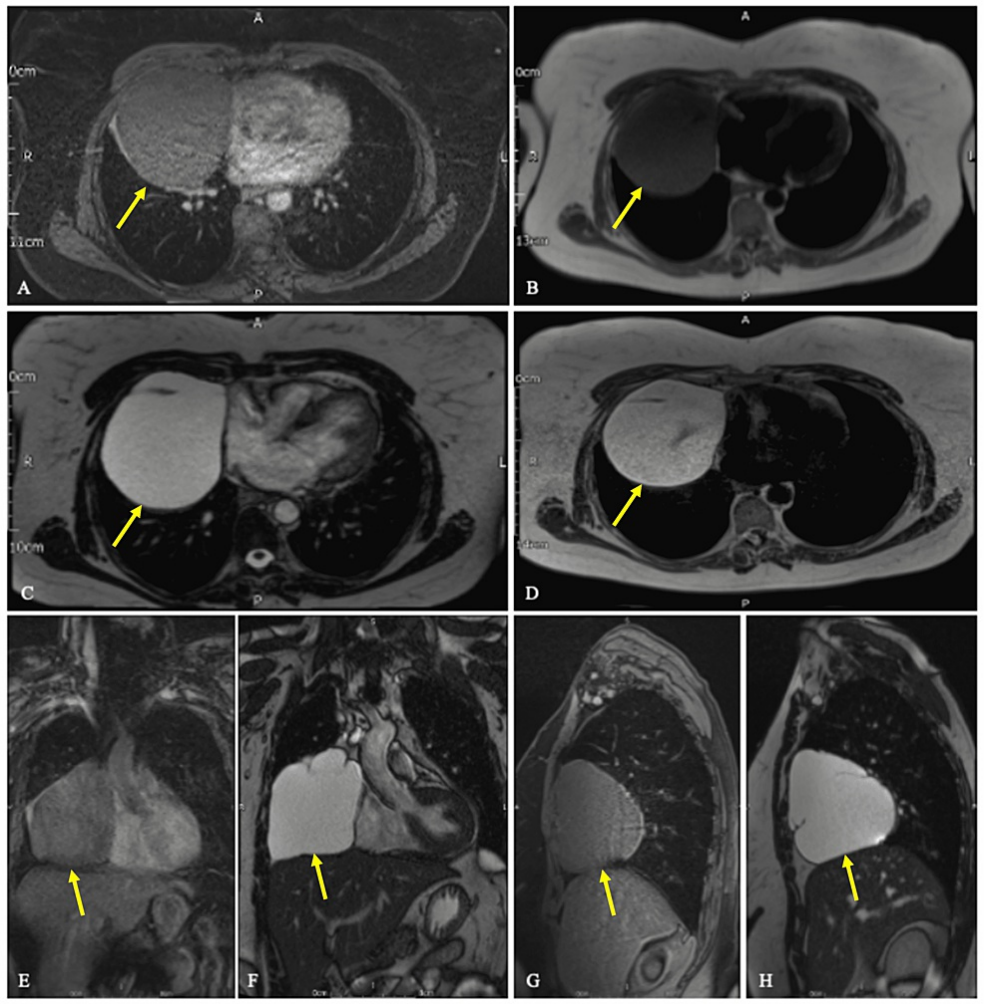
A magnetic resonance imaging (MRI) scan of the chest with gadolinium enhancement. Axial T1-weighted fat-saturated (A) and T1-weighted (B) sequences at the same level showing a water-density lesion with thin walls, located at the cardiophrenic angle (yellow arrow). (C, D) T2-weighted and half-Fourier acquired single-shot turbo-spin echo (HASTE) sequences in axial view at the same level, respectively. (E, F) Coronal T1-weighted and T2-true fast imaging with steady-state-free precession (TRUFI) sequences, respectively. Sagittal T1-weighted (G) and T2-TRUFI (H) sequences.
